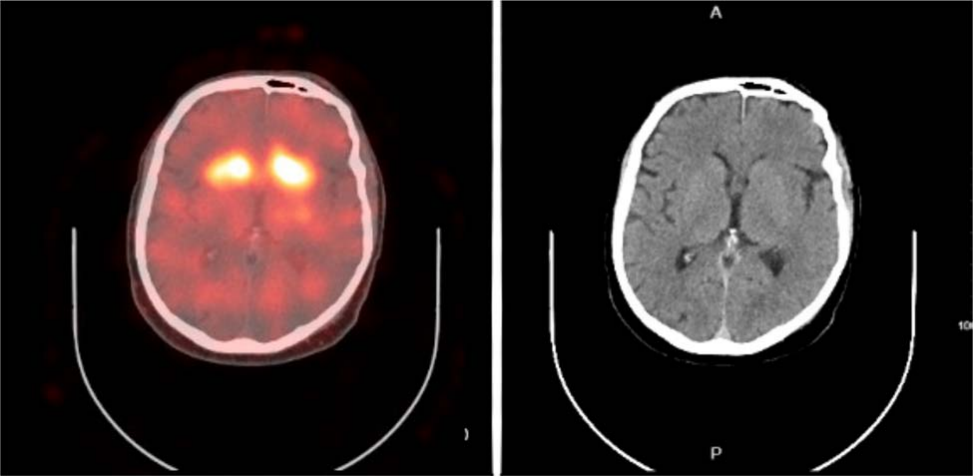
SPECT/CT images show decreased tracer activity in the bilateral putamina.
